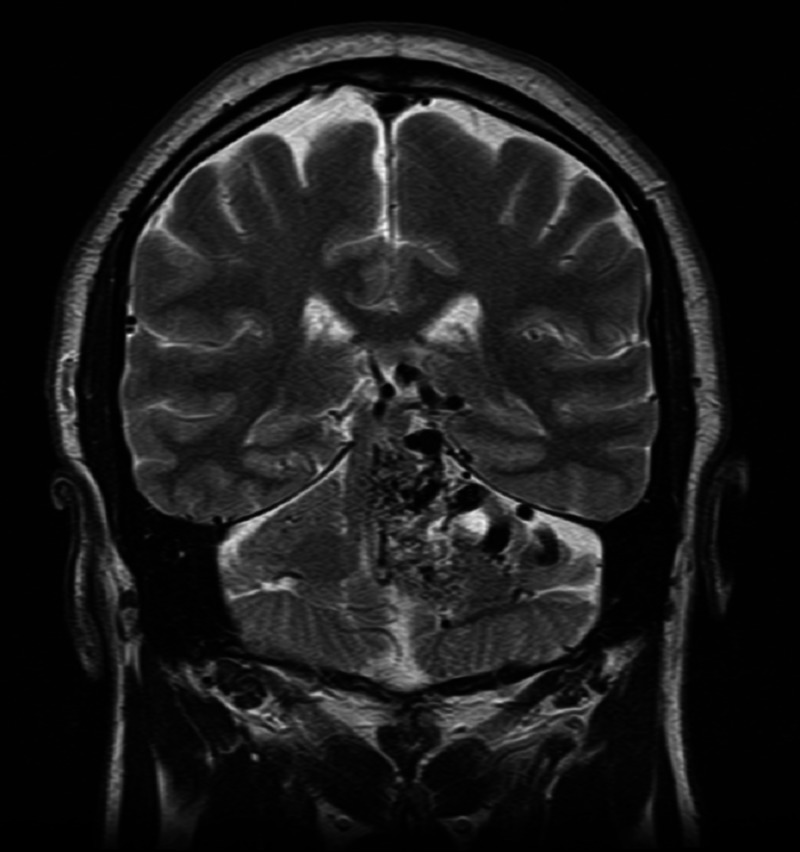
MRI head without contrast, coronal section
